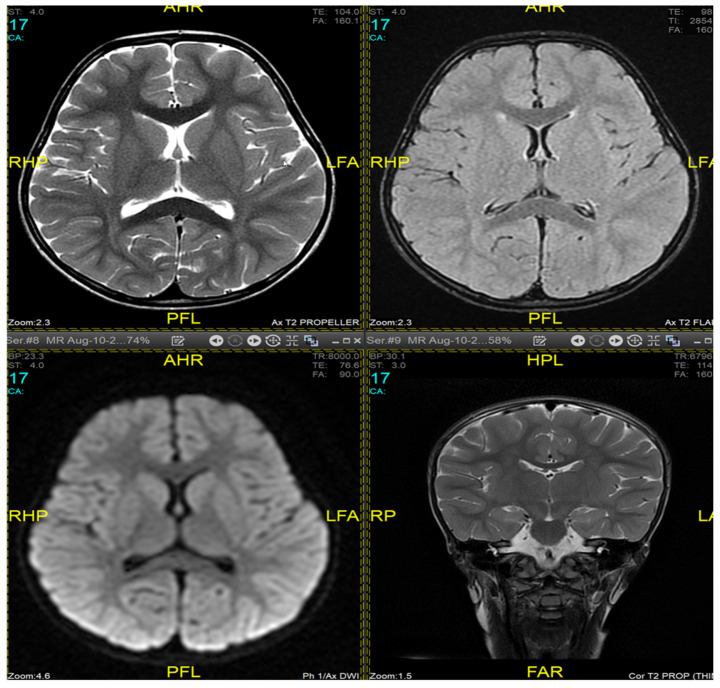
Brain MRI at 2.5 years old (10 August 2017). No evidence of atrophic brain changes or iron deposition.
